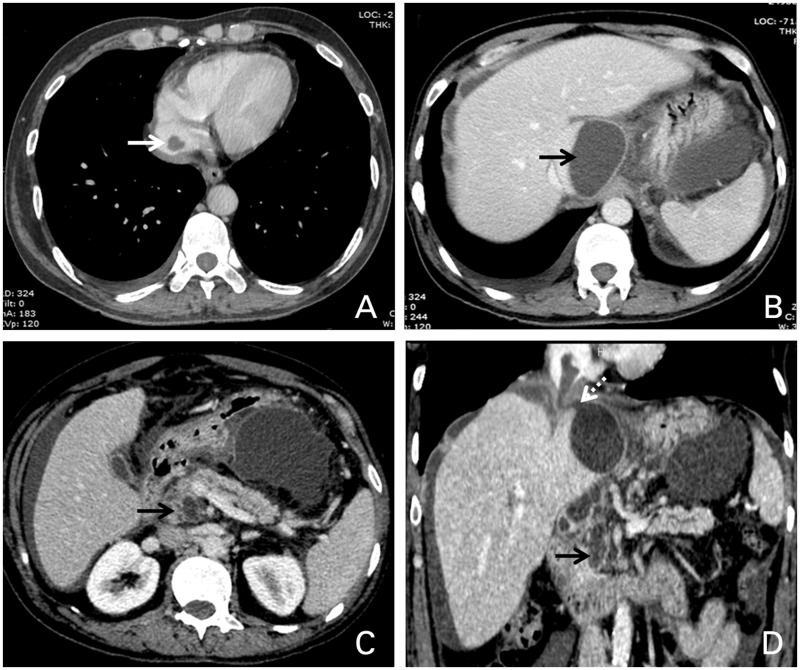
Axial contrast-enhanced CT image showing (A) pseudocyst rupture into the suprahepatic IVC; (B) caudate lobe pseudocyst; (C) pseudocyst in the pancreas. (D) Coronal contrast-enhanced CT image showing well-delineated track (dotted arrow) and pancreatic pseudocyst (arrow).
